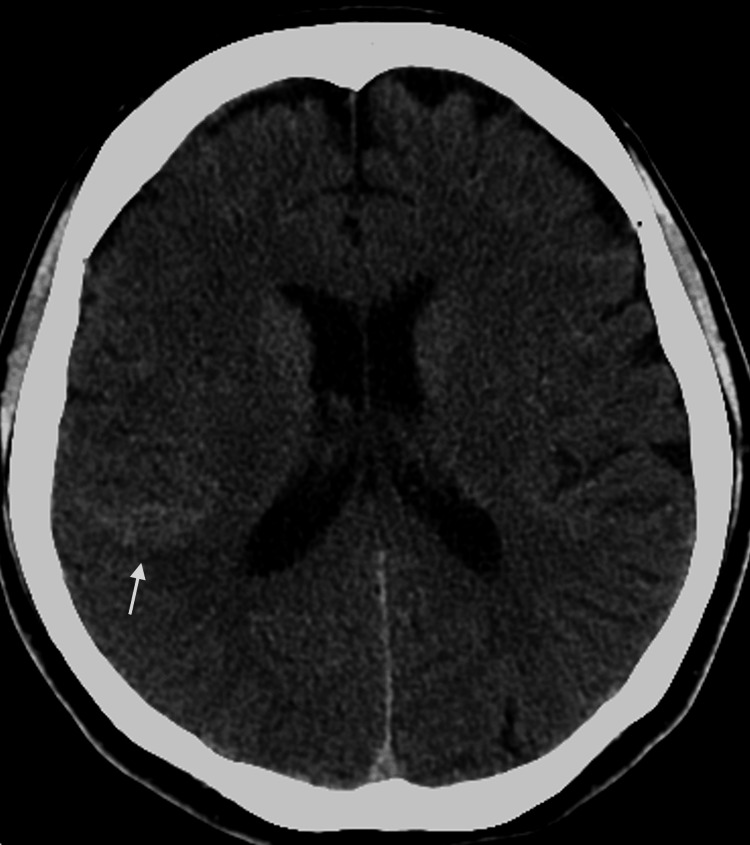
Non-contrast brain CT Non-contrast brain CT demonstrates subarachnoid hemorrhage predominantly located in the right frontotemporal sulci (arrow). CT: Computed tomography
